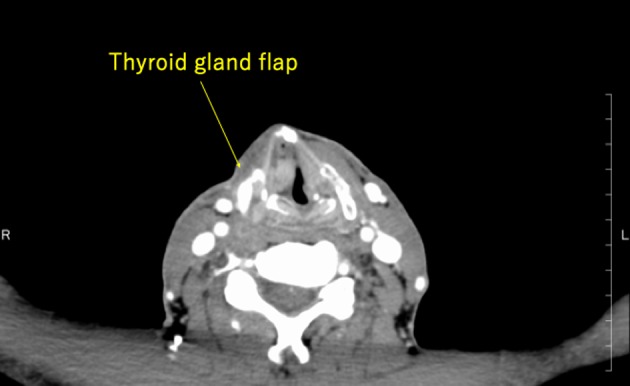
Enhanced CT showing that the thyroid gland flap is accurately placed to cover the defect, and the surgical area is clearly enhanced
